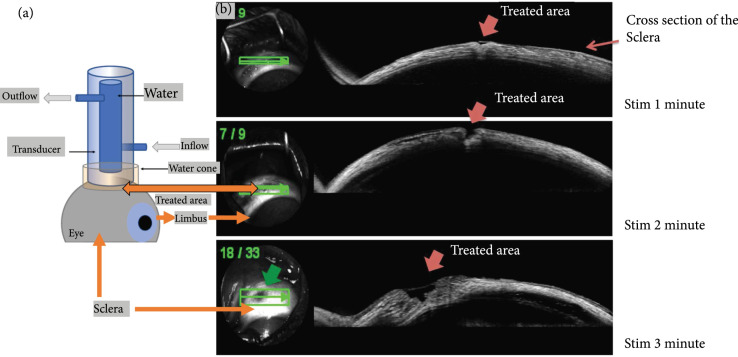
In vivo setup and sample OCT images of treated area. (a) This system allowed us to maintain MIFU intensity where the temperature is compatible with ocular temperature. (b) OCT images of the retina. Top image: sclera was treated with ultrasound for one min. Middle image: sclera was treated with ultrasound for two min. Bottom image: sclera was treated with ultrasound for three min.
